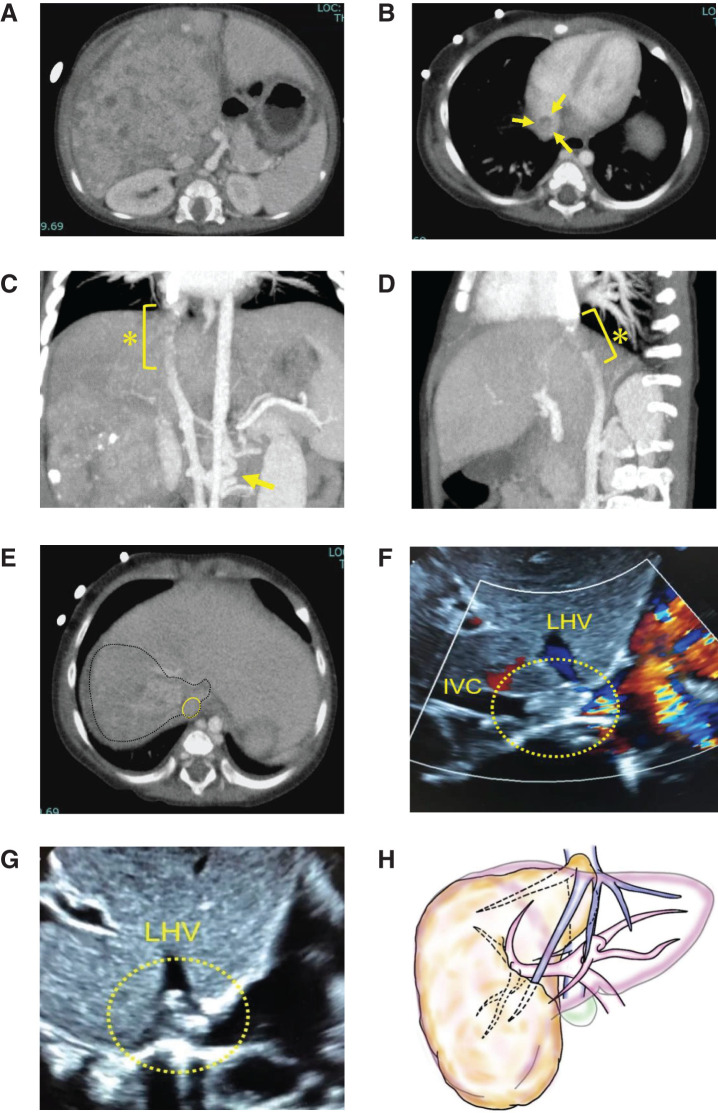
(A) CT revealed a 12-cm hepatic tumor located predominantly in the right lobe. (B) CT scan showing hepatoblastoma with tumor thrombus (arrows) into the IVC close to the right atrium. (C, D) The extent of tumor thrombus in IVC (asterisk) on coronal and sagittal plane CT scan, respectively. The tumor completely occupied from the retrohepatic IVC to the slightly above level of the diaphragm. The developed collateral vein (arrow in [C]) drained into the azygous vein. (E) Relation of the tumor (encircled by black dot line) and the hepatic veins (yellow circle indicated IVC). The tumor had also completely infiltrated the confluence of the LHV. (F, G) The tumor thrombi (yellow dot circle) were confirmed at the confluence of the LHV and IVC by Doppler ultrasound. (H) Hepatoblastoma occupied the entire right lobe and a portion of segment IVa. The tumor invaded into the IVC toward the right atrium, staged as POSTTEXT III (P1, V3, M0). IVC, inferior vena cava; LHV, left hepatic vein
